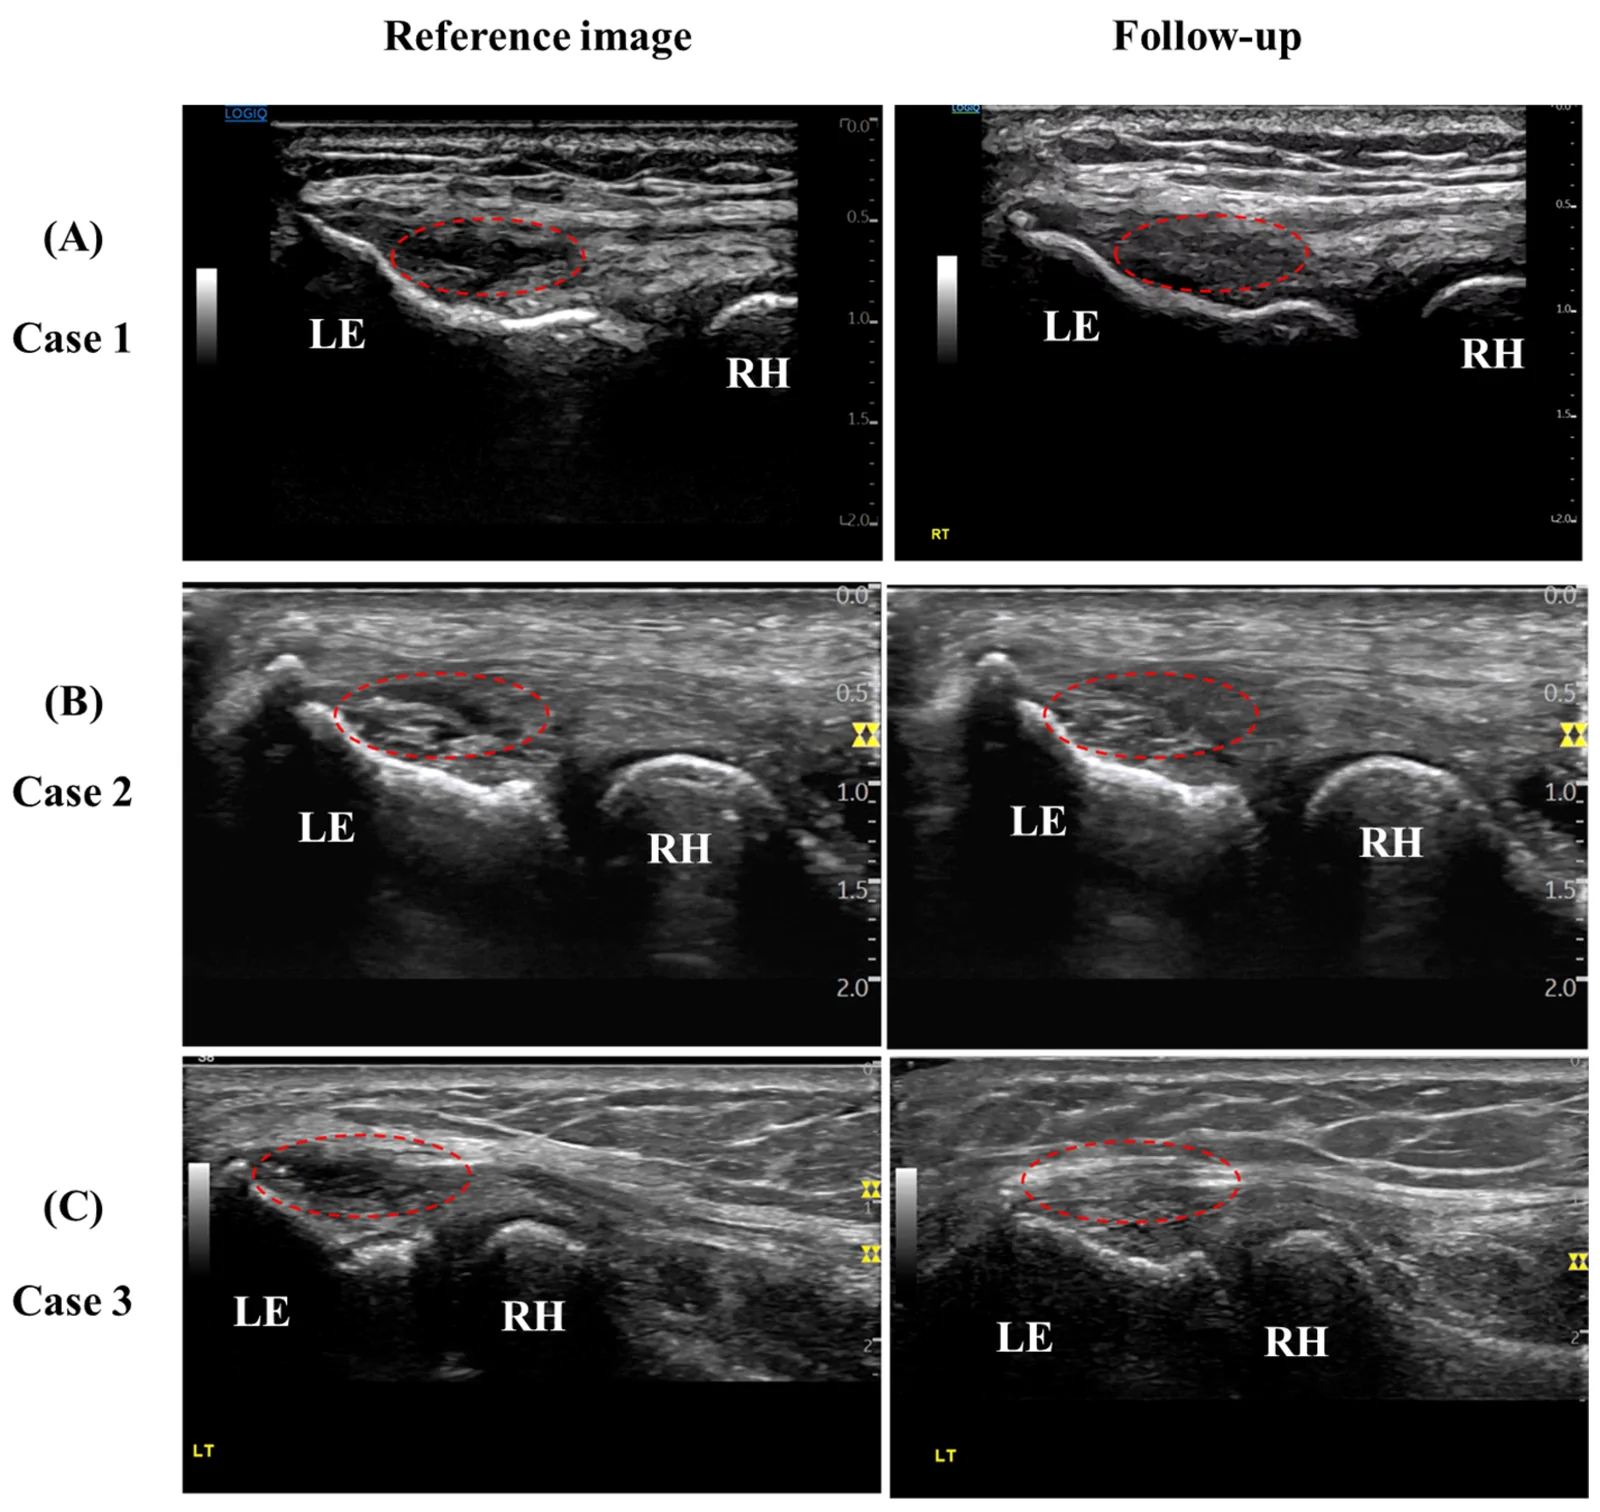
Longitudinal ultrasound images of the common extensor tendon at the lateral elbow: pre-treatment (Cases 1 and 2) or at treatment completion (Case 3)/(left) and follow-up (right). (A) Case 1. (Left): Hypoechoic partial tear at the CET enthesis with disrupted fibrillar echotexture. (Right): Follow-up at 114 days after treatment completion, showing increased echogenicity. (B) Case 2. (Left): Hypoechoic partial tear at the CET enthesis with bony irregularity at the lateral epicondyle. (Right): Follow-up at 103 days after treatment completion, showing partial resolution of the hypoechoic defect. (C) Case 3. (Left): Residual hypoechoic changes at the CET at treatment completion. (Right): Follow-up at 270 days after treatment completion, showing marked reduction in hypoechoic area with near-complete restoration of fibrillar echotexture. Image interpretation was performed by the treating practitioner, who was not blinded to treatment stage or clinical outcome. CET, common extensor tendon. LE, lateral epicondyle; RH, radial head.
